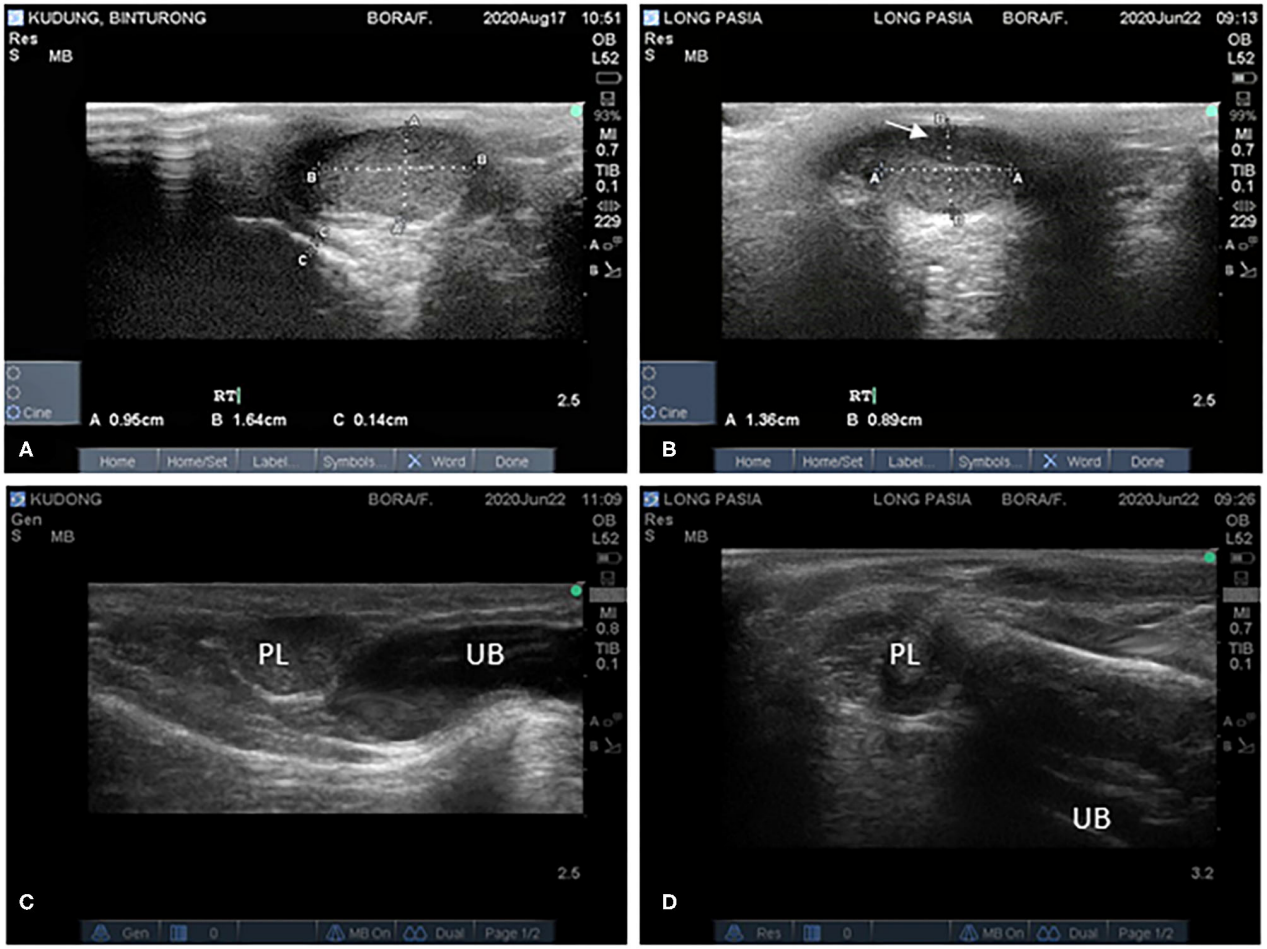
Ultrasonographic images of testes of (A) Binturong 1 and (B) Binturong 2 (white arrow pointing at hypoechoic structure). Ultrasonographic images of reproductive tracts of (C) Binturong 1 (oval-shaped prostatic lobe, PL, with uniform echogenicity) and (D) Binturong 2 (heterogenous echotexture of PL). UB, Urine bladder.
